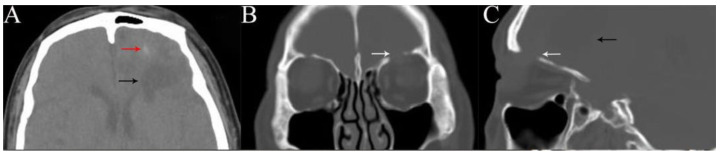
Head computed tomography (CT) scan showed a short, strip-like, slightly high-density shadow (red arrow) and a cystic mass (black arrow) in the left frontal lobe, and a fracture of the left superior orbital wall (white arrow). Axial (A), coronal (B), and sagittal views (C).
